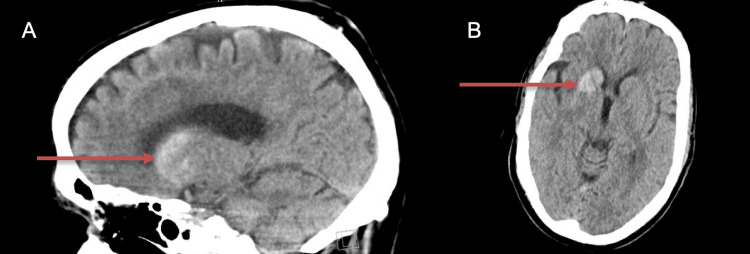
Head CT without contrast in sagittal (Panel A) and transverse views (Panel B). Noted hyperdense lesion (red arrow) in the right basal ganglia and anterior limb of the right internal capsule.
